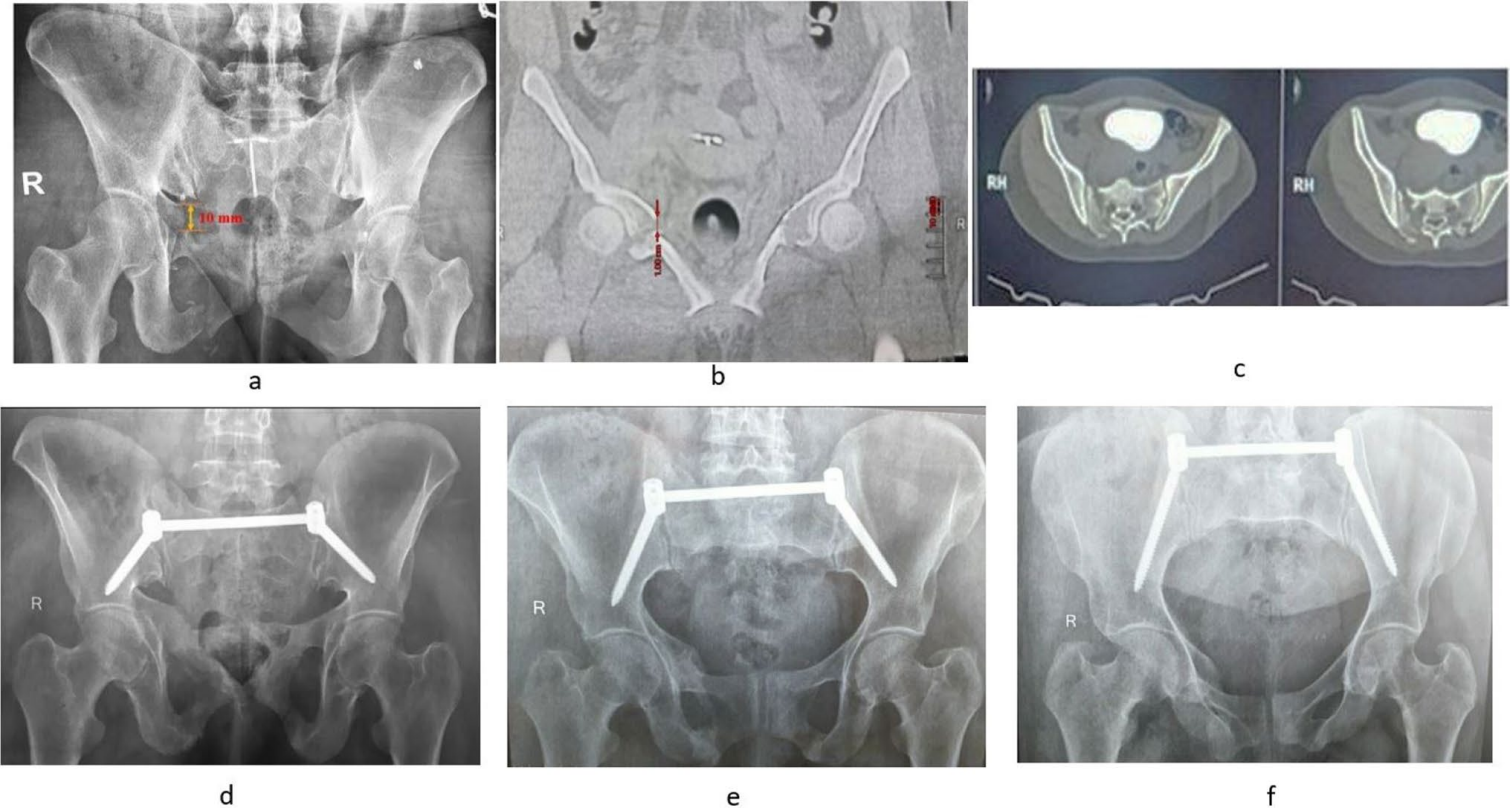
A LC 1 case with posterior-only fixation; measuring of initial displacement at the rami in preoperative AP X-ray (a) and CT (b), c axial CT cut, follow-up at one year is shown in the outlet (d), AP e and inlet f views. The patient healed with no secondary displacement
